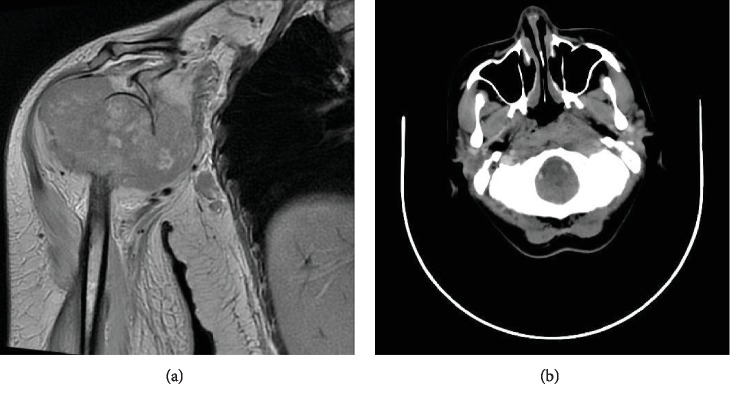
(a) MRI of right upper extremities showed malignant bone tumour. (b) CT scan of nasopharynx showed mass and bilateral lymphadenopathy.
